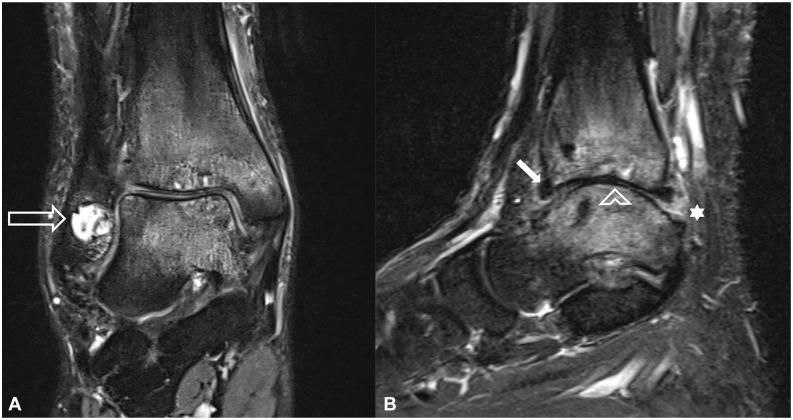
MRIs before fluoroscopy-guided intraarticular injection of an ankle joint in a 40-year-old female patient shown on (A) coronal PD Dixon fat suppressed and (B) sagittal STIR. MRIs show severe bone marrow edema, subchondral cyst formation (open arrow), moderate cartilage damage (arrowhead) and soft tissue edema (asterisk), and little osteophyte formation (arrow). Patient responded very well to treatment, with a decline in VAS score from 5 before to 0 after injection (100% decline). MRIs, magnetic resonance images; PD, proton density; STIR, short tau inversion recovery; VAS, visual analog scale.
